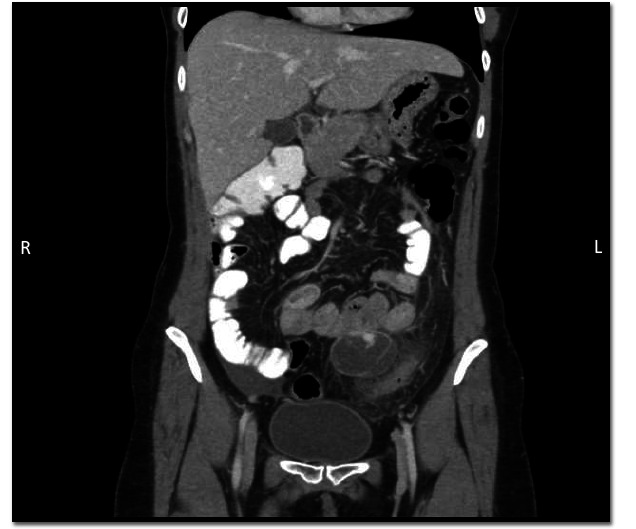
Coronal-plane CT of abdomen and pelvis demonstrating a hypodense lesion located left of the midline of the abdomen containing high-density material suggestive of hemorrhage. This lesion appeared inseparable from the small bowel and was surrounded by inflammatory changes. The adjacent colon showed mild thickening.
